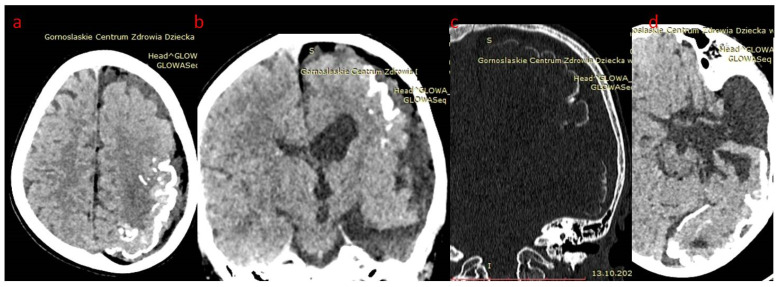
(a–d) Patient 1. Head CT brain window: (a) axial and (b) reformatted coronal plane; hemiatrophy of left hemisphere, left ventriculomegaly, meningeal lipomatosis, arachnoid cyst in temporal fossa and gyral calcifications; (c) bone window coronal reformatted plane: extensive left sided gyral calcifications; (d) axial plain—left arachnoid cyst.
